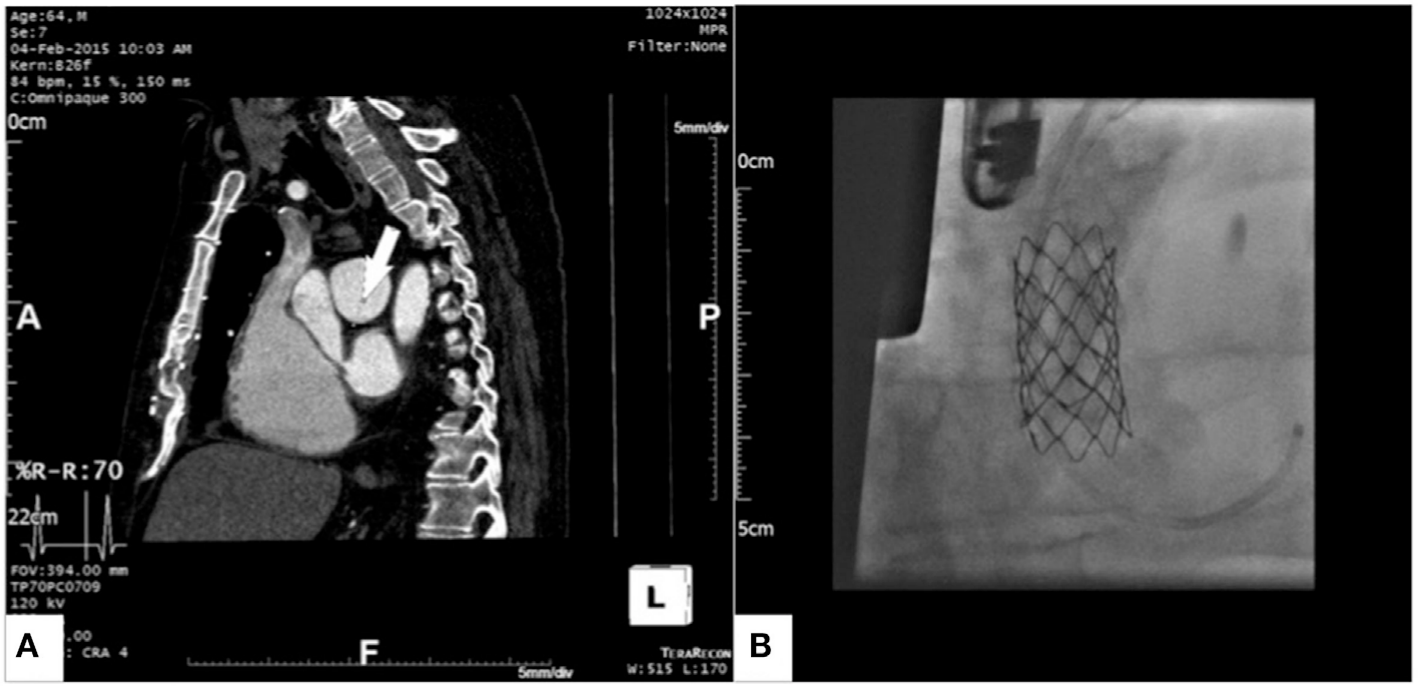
Gated computed tomography scan showing severe stenosis at the left atrial end of the pericardial baffle [(A), arrow] and 16 mm × 45 mm Premounted CP Stent expanded within the pericardial baffle [(B), the sheath can be seen in the stump of the superior vena cava].
